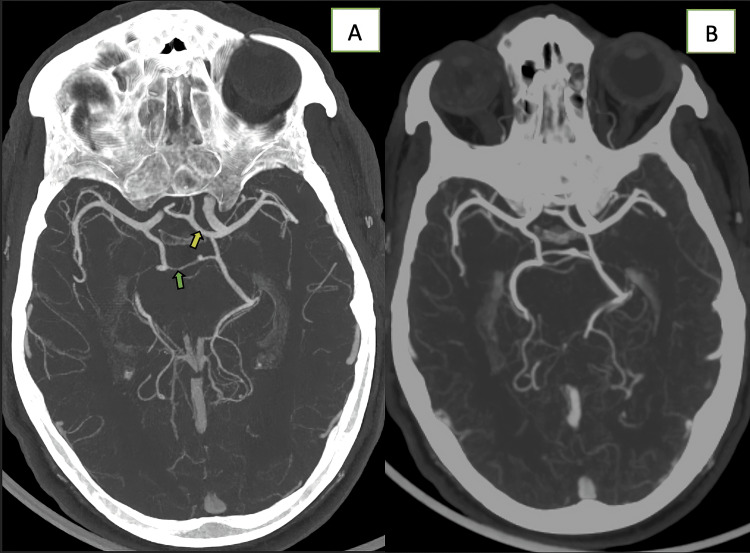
CT angiography of the head and neck does not show any hemodynamically significant stenosis or thrombosis but reveals a fetal-type right PCA (green arrow) and agenesis of the right A1 segment, with both anterior cerebral arteries arising from a single trunk (yellow arrow) (A). The rest of the circle of Willis is normal (B). PCA: posterior cerebral artery
